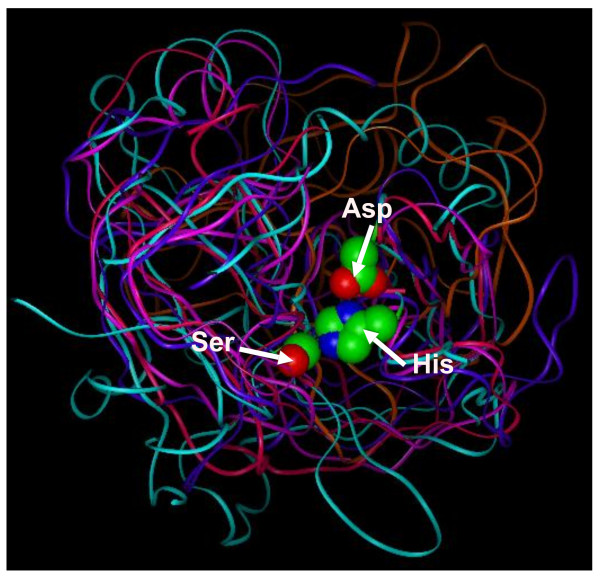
Superposed structures of X-ray and modeled structures of the selected proteases of the PA clan. Structures of the protozoan (*Plasmodium falciparum*, magenta), archaeon (*Pyrococcus furiosus*, cyan), fungus (*Neurospora crassa*, purple) and plant (*Arabidopsis thaliana*, fuchsia pink) PA proteases were superposed with the human x-ray structure (1SGI, *Homo sapiens*, orange). The catalytic triad residues (His, Asp, Ser) are shown in ball and stick models.
